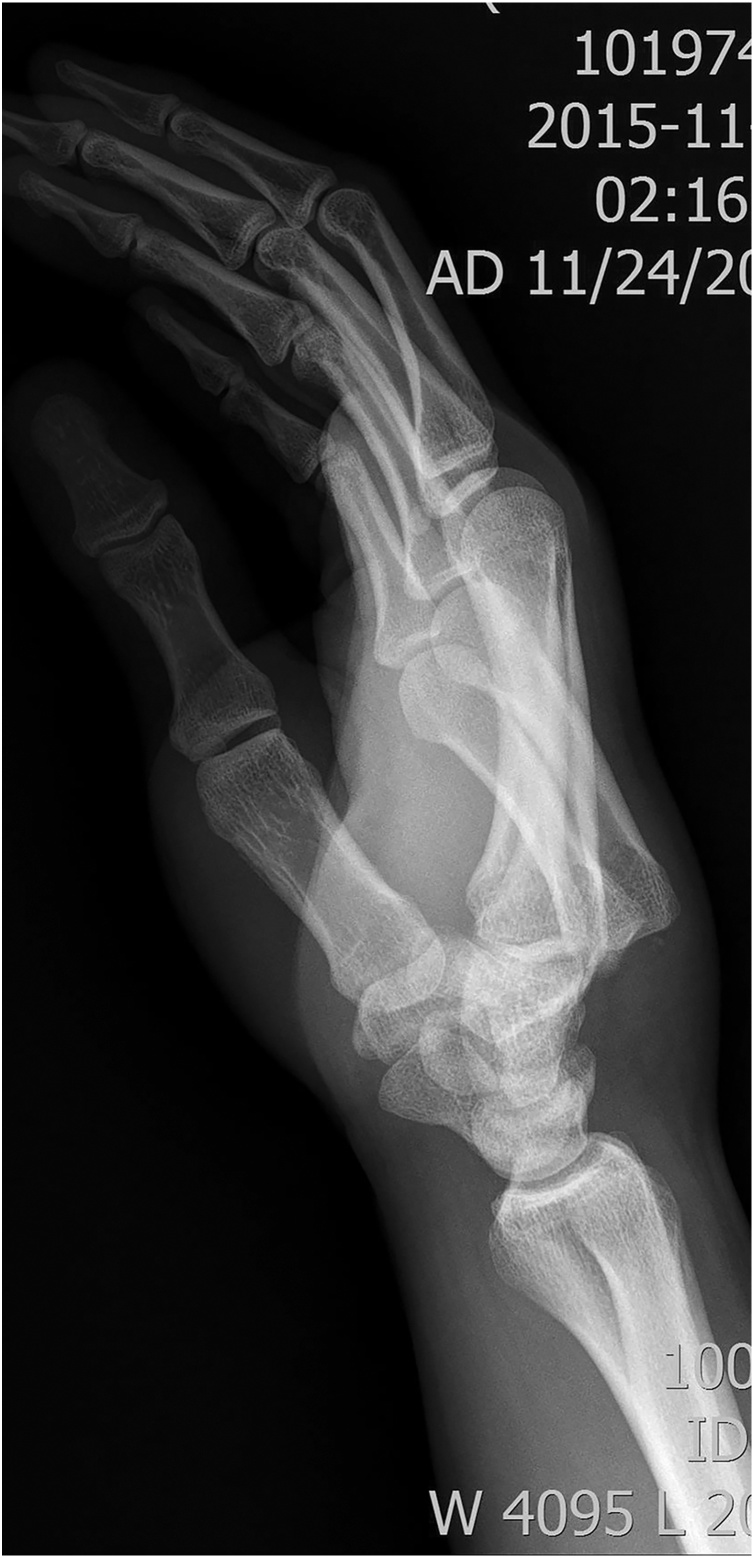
Pre-operative X-ray (lateral view). X-ray demonstrate a posterior dislocation of the 4th and 5th metacarpal bases in CMC joint, with fractured tiny bone fragments in the ventral side of the CMC joint.
